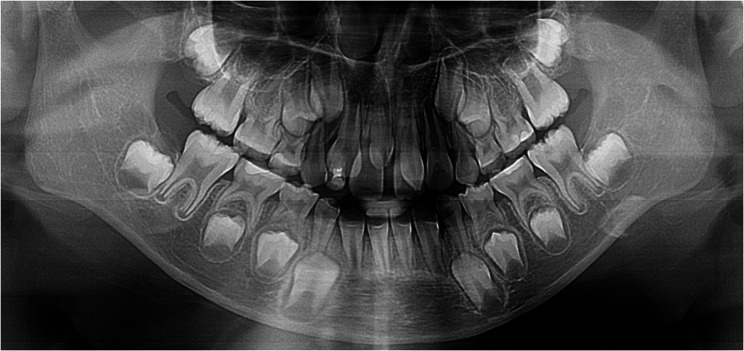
Panoramic radiograph of a 7-year-old female showing a congenitally missing bilateral maxillary second premolars, 2 supernumerary teeth located in the maxillary anterior region
